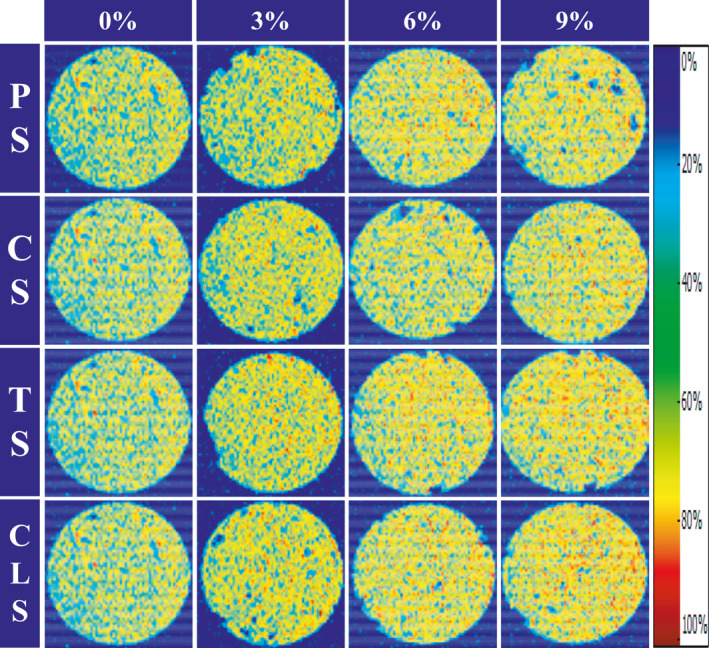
MRI images for shrimp surimi with different types of starch added, PS, potato starch; CS, corn starch; TS, tapioca starch; CLS, cross‐linked starch
